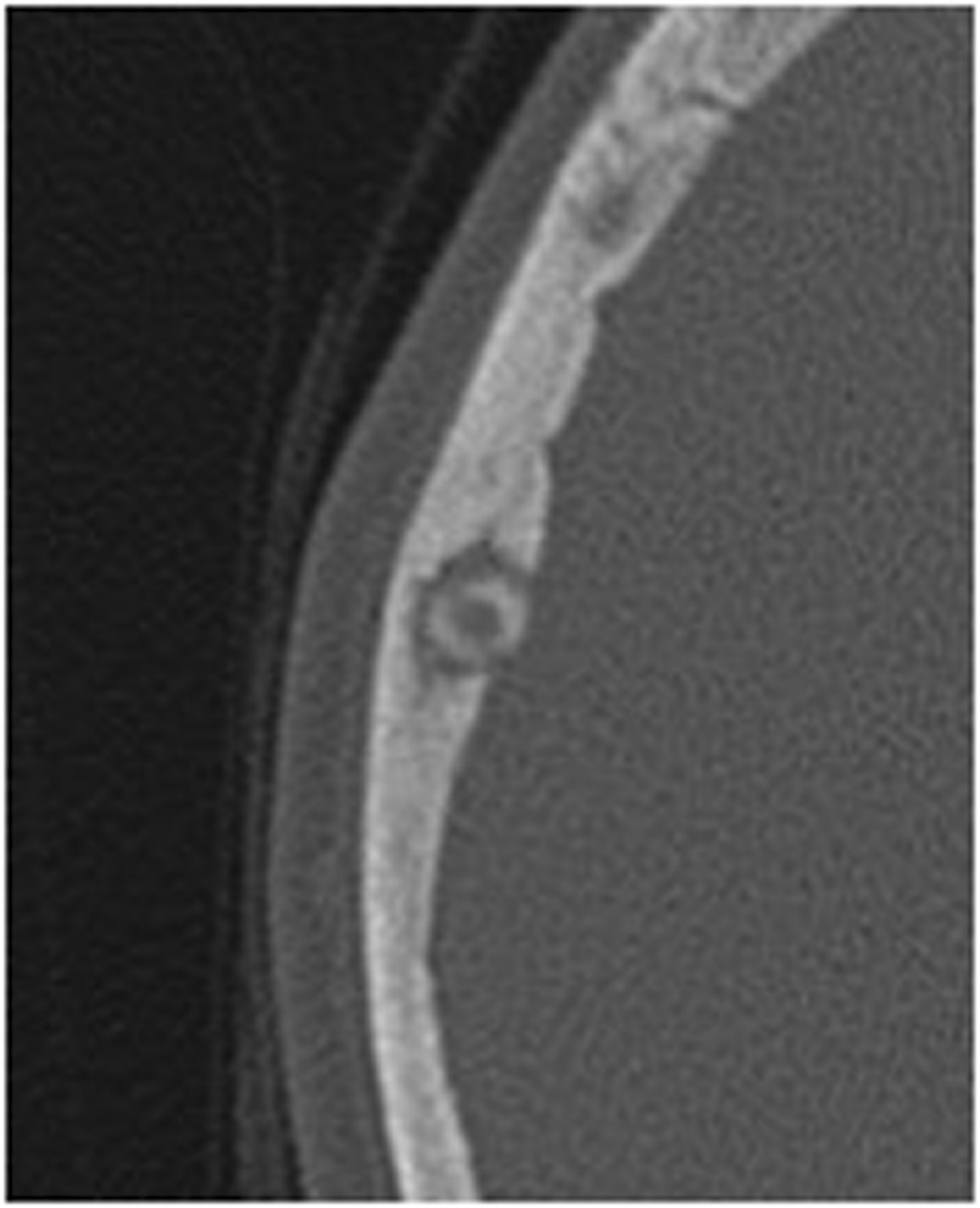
Axial bone window CT image shows a small rounded well‐defined lytic lesion surrounded by sclerosis and expansion of the diploic space in the right parietal area
